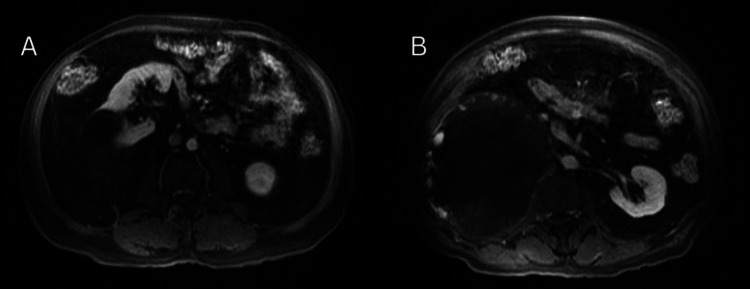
Axial MRI of Adrenal Hemangioma Axial MRI T1-weighted (A) and post-contrast sequences with fat saturation (B) showing a large hypointense retroperitoneal mass with peripheral nodular enhancement. The adrenal origin is favored. The imaging appearance, while nonspecific, remained concerning for a primary adrenal malignancy.
